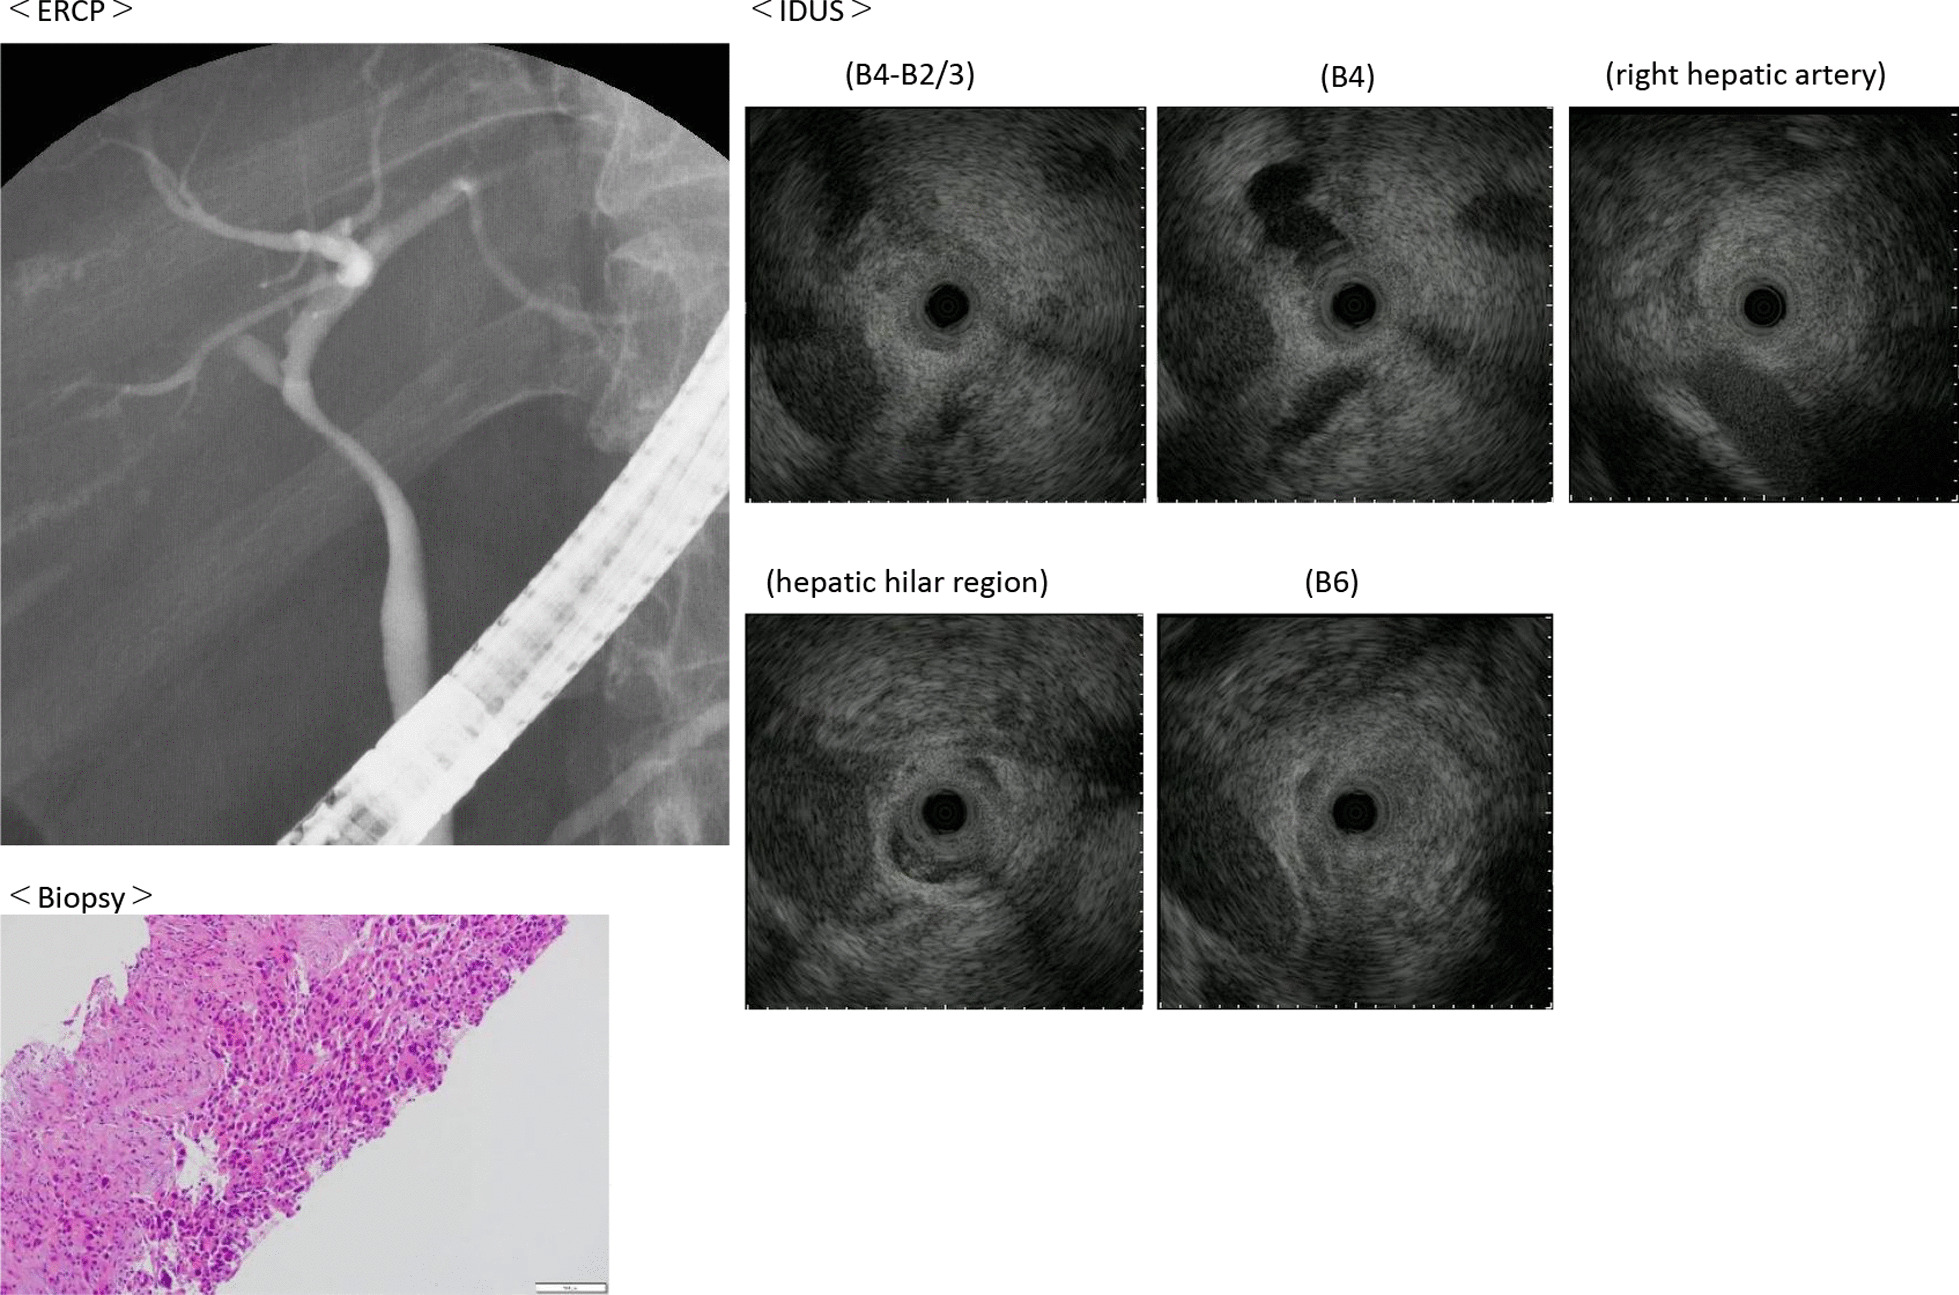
Endoscopic retrograde cholangiopancreatography revealed displacement of the upper bile duct. Intraductal ultrasonography showed irregular wall thickening and disappearance of the wall structure in bile ducts from the B4 branch to distal B2 and B3. Percutaneous transhepatic biliary biopsy revealed a poorly differentiated carcinoma.
